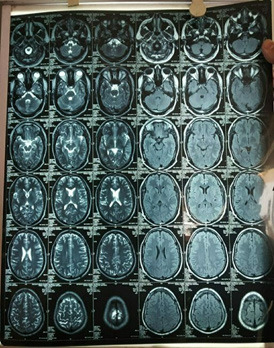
MRI of brain showing T2 sagittal and coronal images showing subacute infraction in parietoccipital region.
